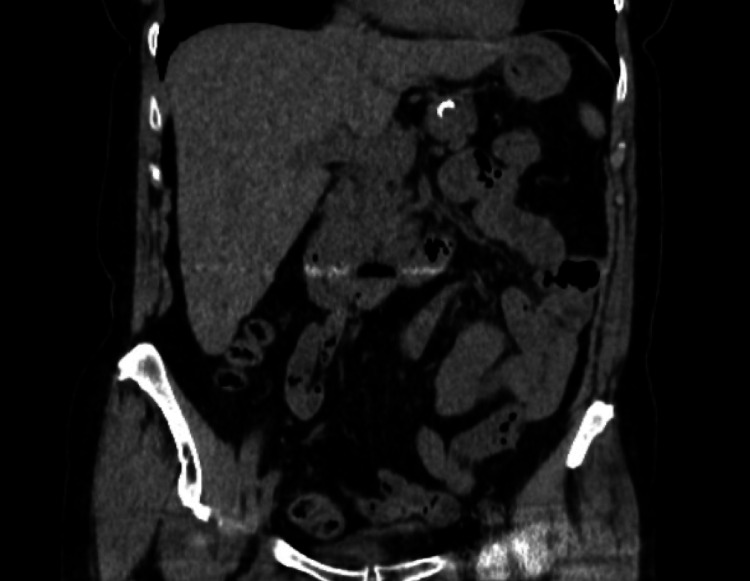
CT abdomen and pelvis showing thickening of the descending colon along with peri-colic fat stranding consistent with acute colitis
